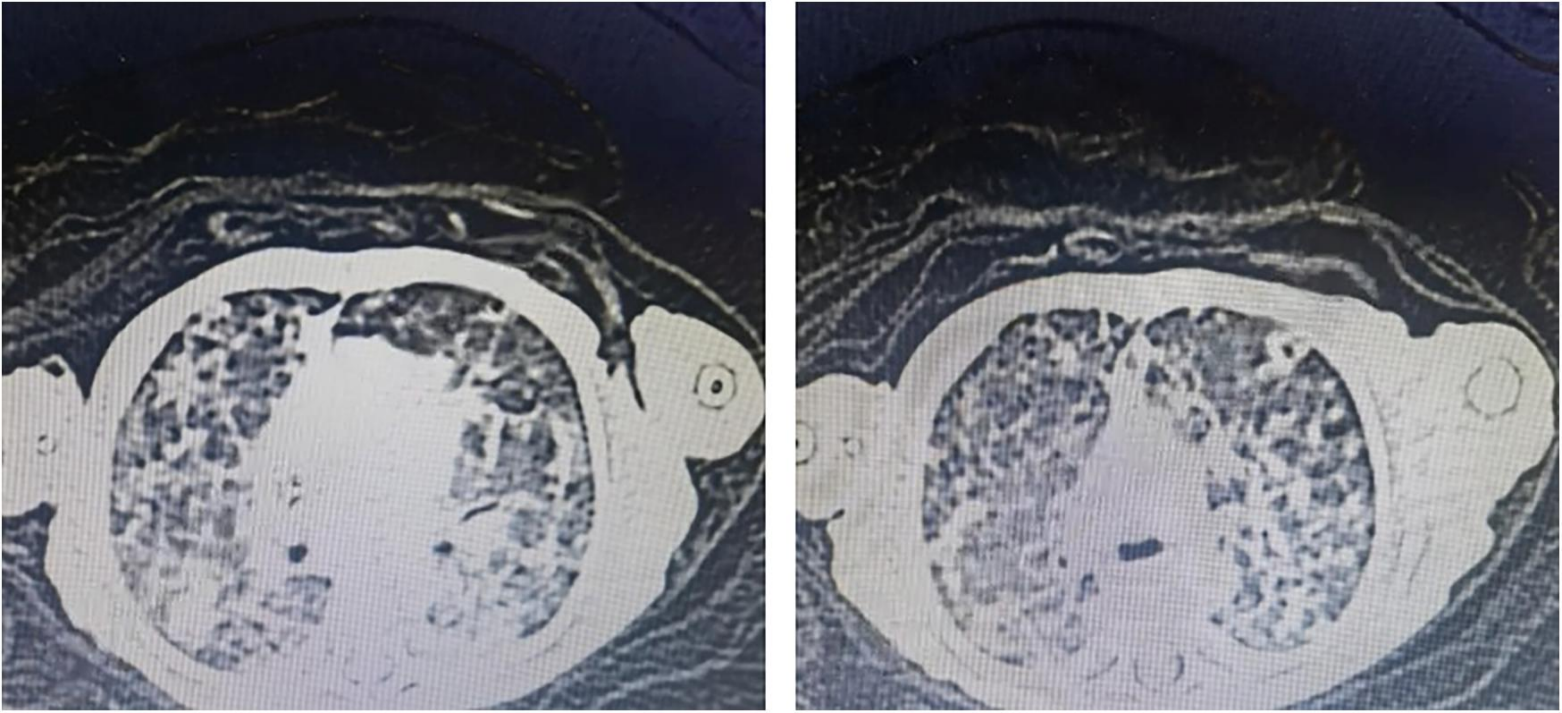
CT image showing miliary TB.
